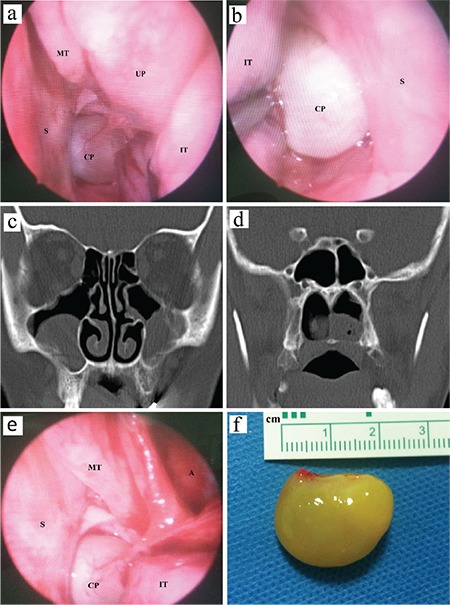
Endoscopic view of the left nasal cavity (a) and the right nasal cavity (b), Coronal CT view of the sinuses demonstrating bilateral maxillary opacification (c) and soft-tissue opacification in the nasopharynx (d), During endoscopic surgery, view of the choanal polyp originating from maxillary sinus ostium mucosa (e), Macroscopic appearance of the solitary polyp (f).
